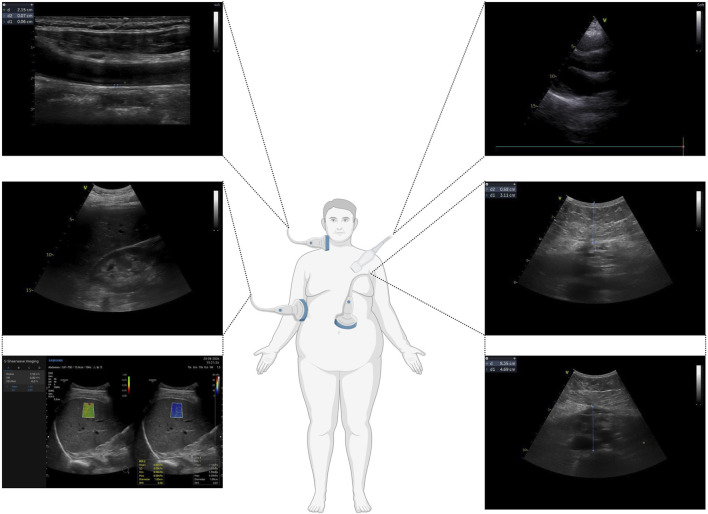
Proposed multiparamatric ultrasound protocol for CRMS assessment. Probe placement areas and representative images. From top on the left: carotid artery, hepatic parenchyma - kidney cortex interface, shear wave elastography of the liver. From top on the right: parasternal long axis of the heart, abdominal wall layers thickness measurement, omental fat thickness measurement.
